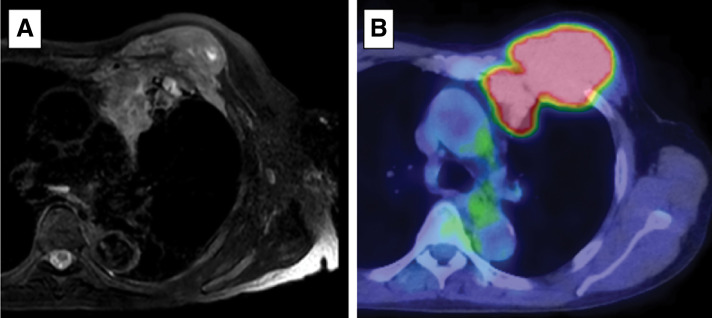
Preoperative MRI and FDG-PET. (A) Axial MRI section of a fat-suppressed T2-weighted sequence showing the left CW and an anterior mildly hyperintense signal. (B) Axial FDG-PET section overlaid on the CT scan showing the left CW tumor’s high glucose uptake, with a maximum standardized uptake value of 16.0. CW, chest wall; FDG, fluorodeoxyglucose
